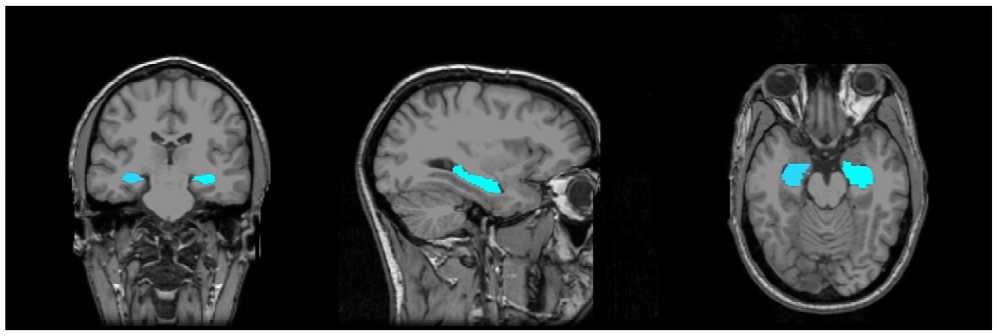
Freesurfer hippocampal region of interest. Coronal, sagittal, and axial (1.5T) T1-weighted MR images from the Freesurfer Image Analysis Suite of sample subject “Bert”, with color overlays depicting the extent of the Freesurfer hippocampal region of interest used to derive hippocampal volumes.
